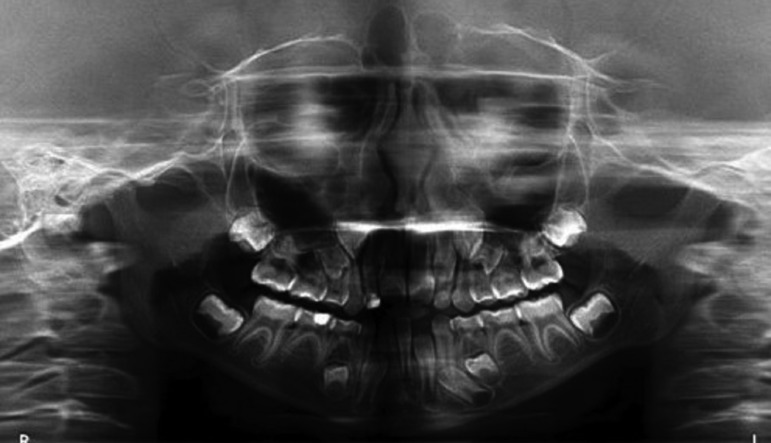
Panoramic radiograph of patient #4 of this study. Agenesis of the permanent maxillary and mandibular second premolars and permanent mandibular lateral incisor was observed.
